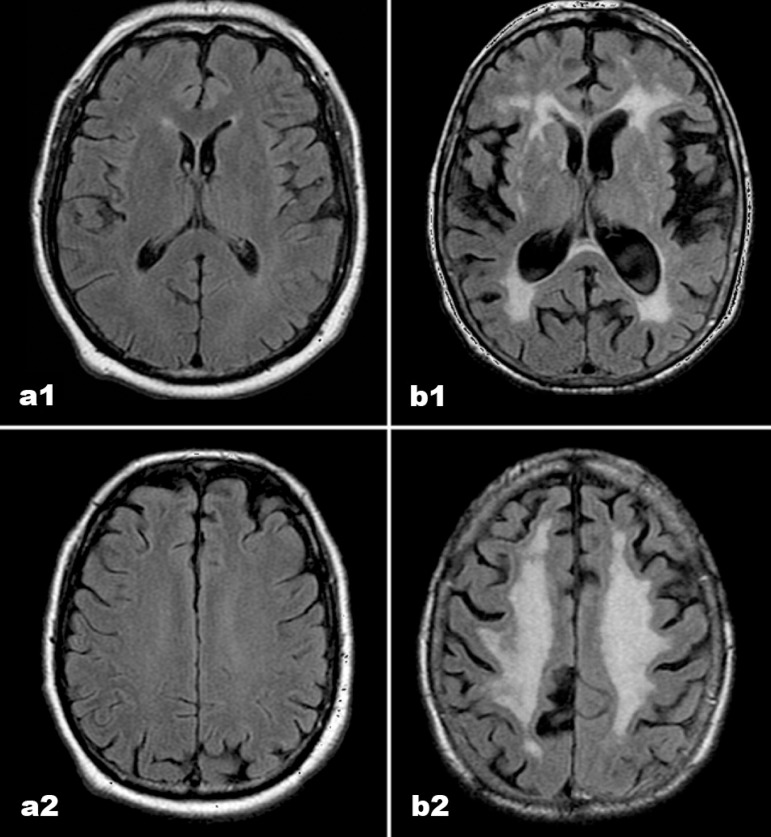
MR scans in FLAIR acquisition (axial plane) – sections at basal
